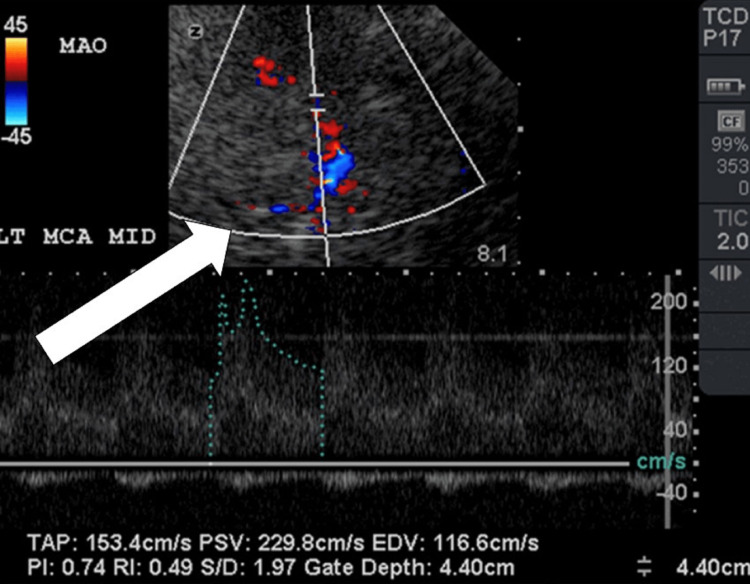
TCD on POD 7 showing moderate vasospasm of the MCA. MCA: middle cerebral artery; POD: postoperative day; TCD: transcranial doppler.
